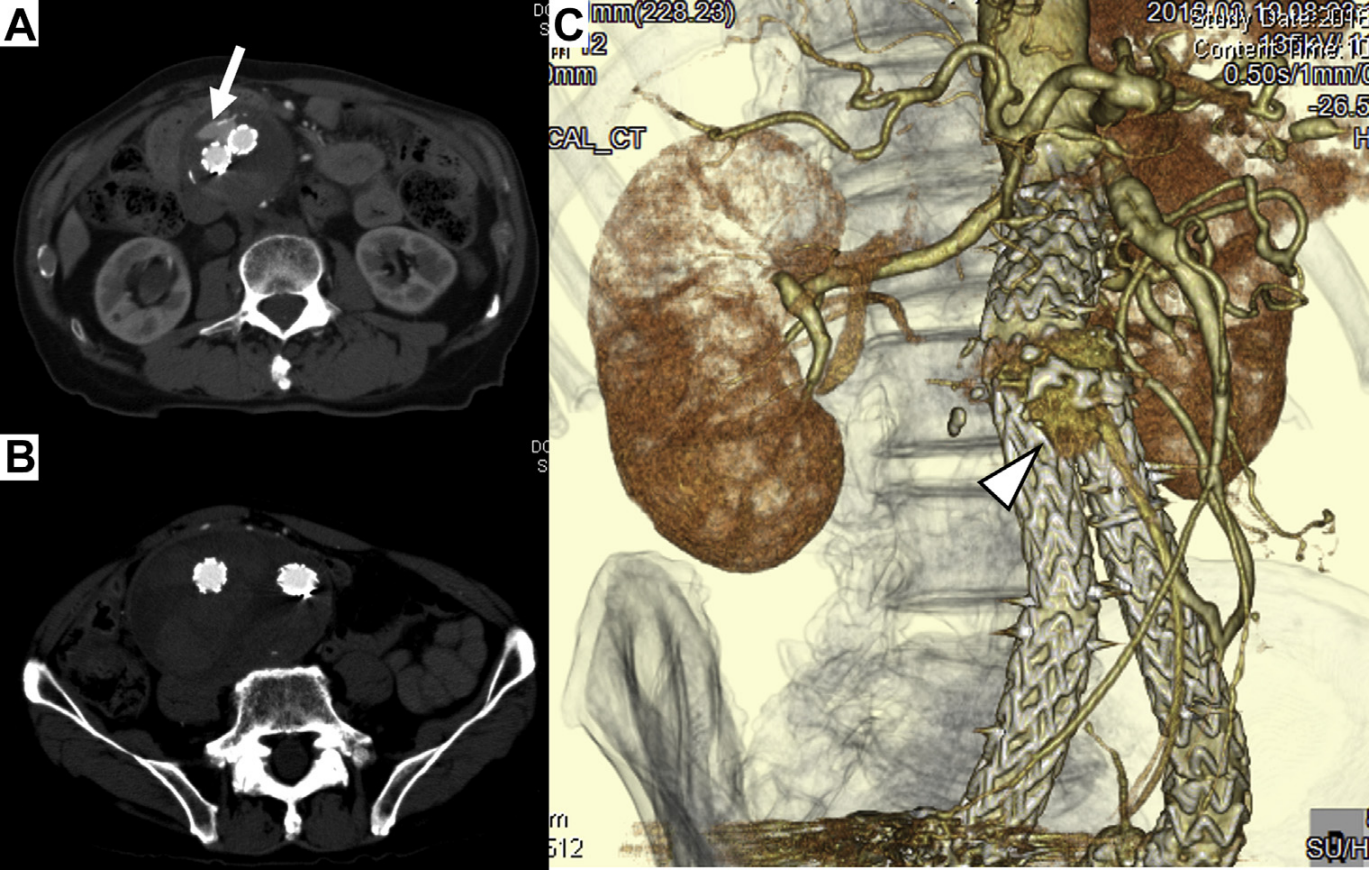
Computed tomography (CT) angiogram at the present admission (4 months after the first operation). CT scan revealing type II endoleak (*arrow*; A), rapid dilatation of the aneurysm sac (maximum transverse diameter had increased by 15 mm after first operation) and spontaneous obstruction of the aortocaval fistula (ACF; B). C, Three-dimensional CT angiogram showing disappearance of ACF (*open arrowhead* indicates type II endoleak via a meandering mesenteric artery).
